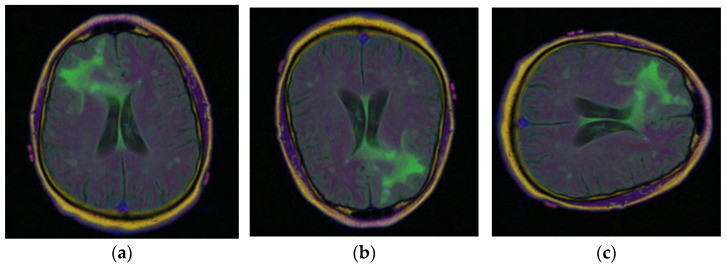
Samples of Brain MRI Images [31]: (a) Original Image, (b) Vertical Flip, (c) Horizontal Flip.
